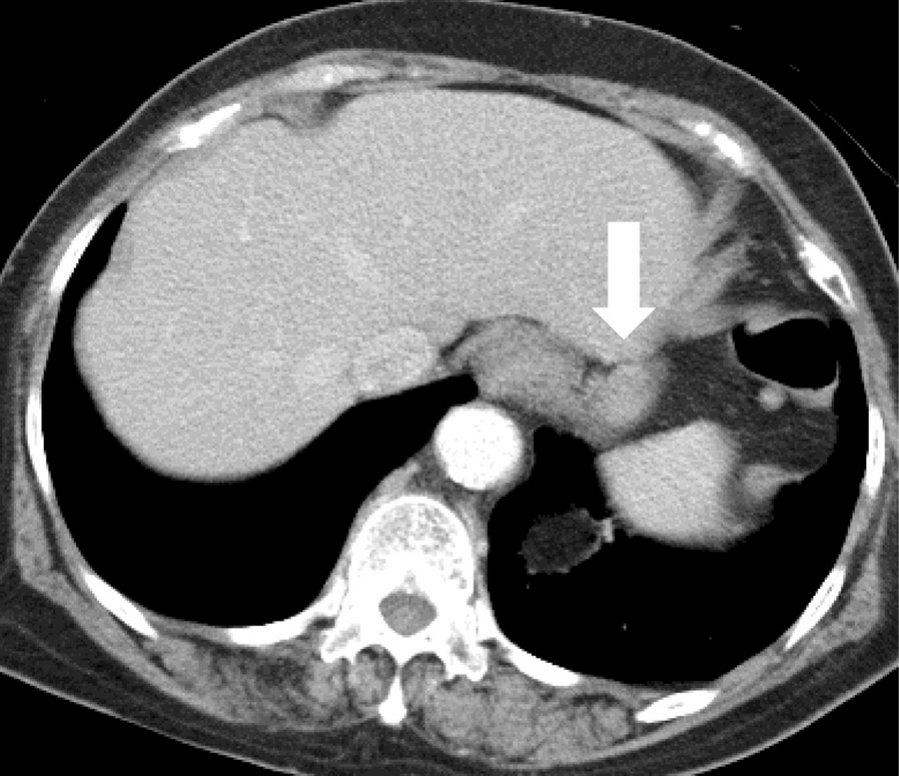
Computed tomography scan reveals an iso-enhancing and well-defined nodule (approximately 16 × 13 mm) protruding from the upper edge at the fornix (arrow)
